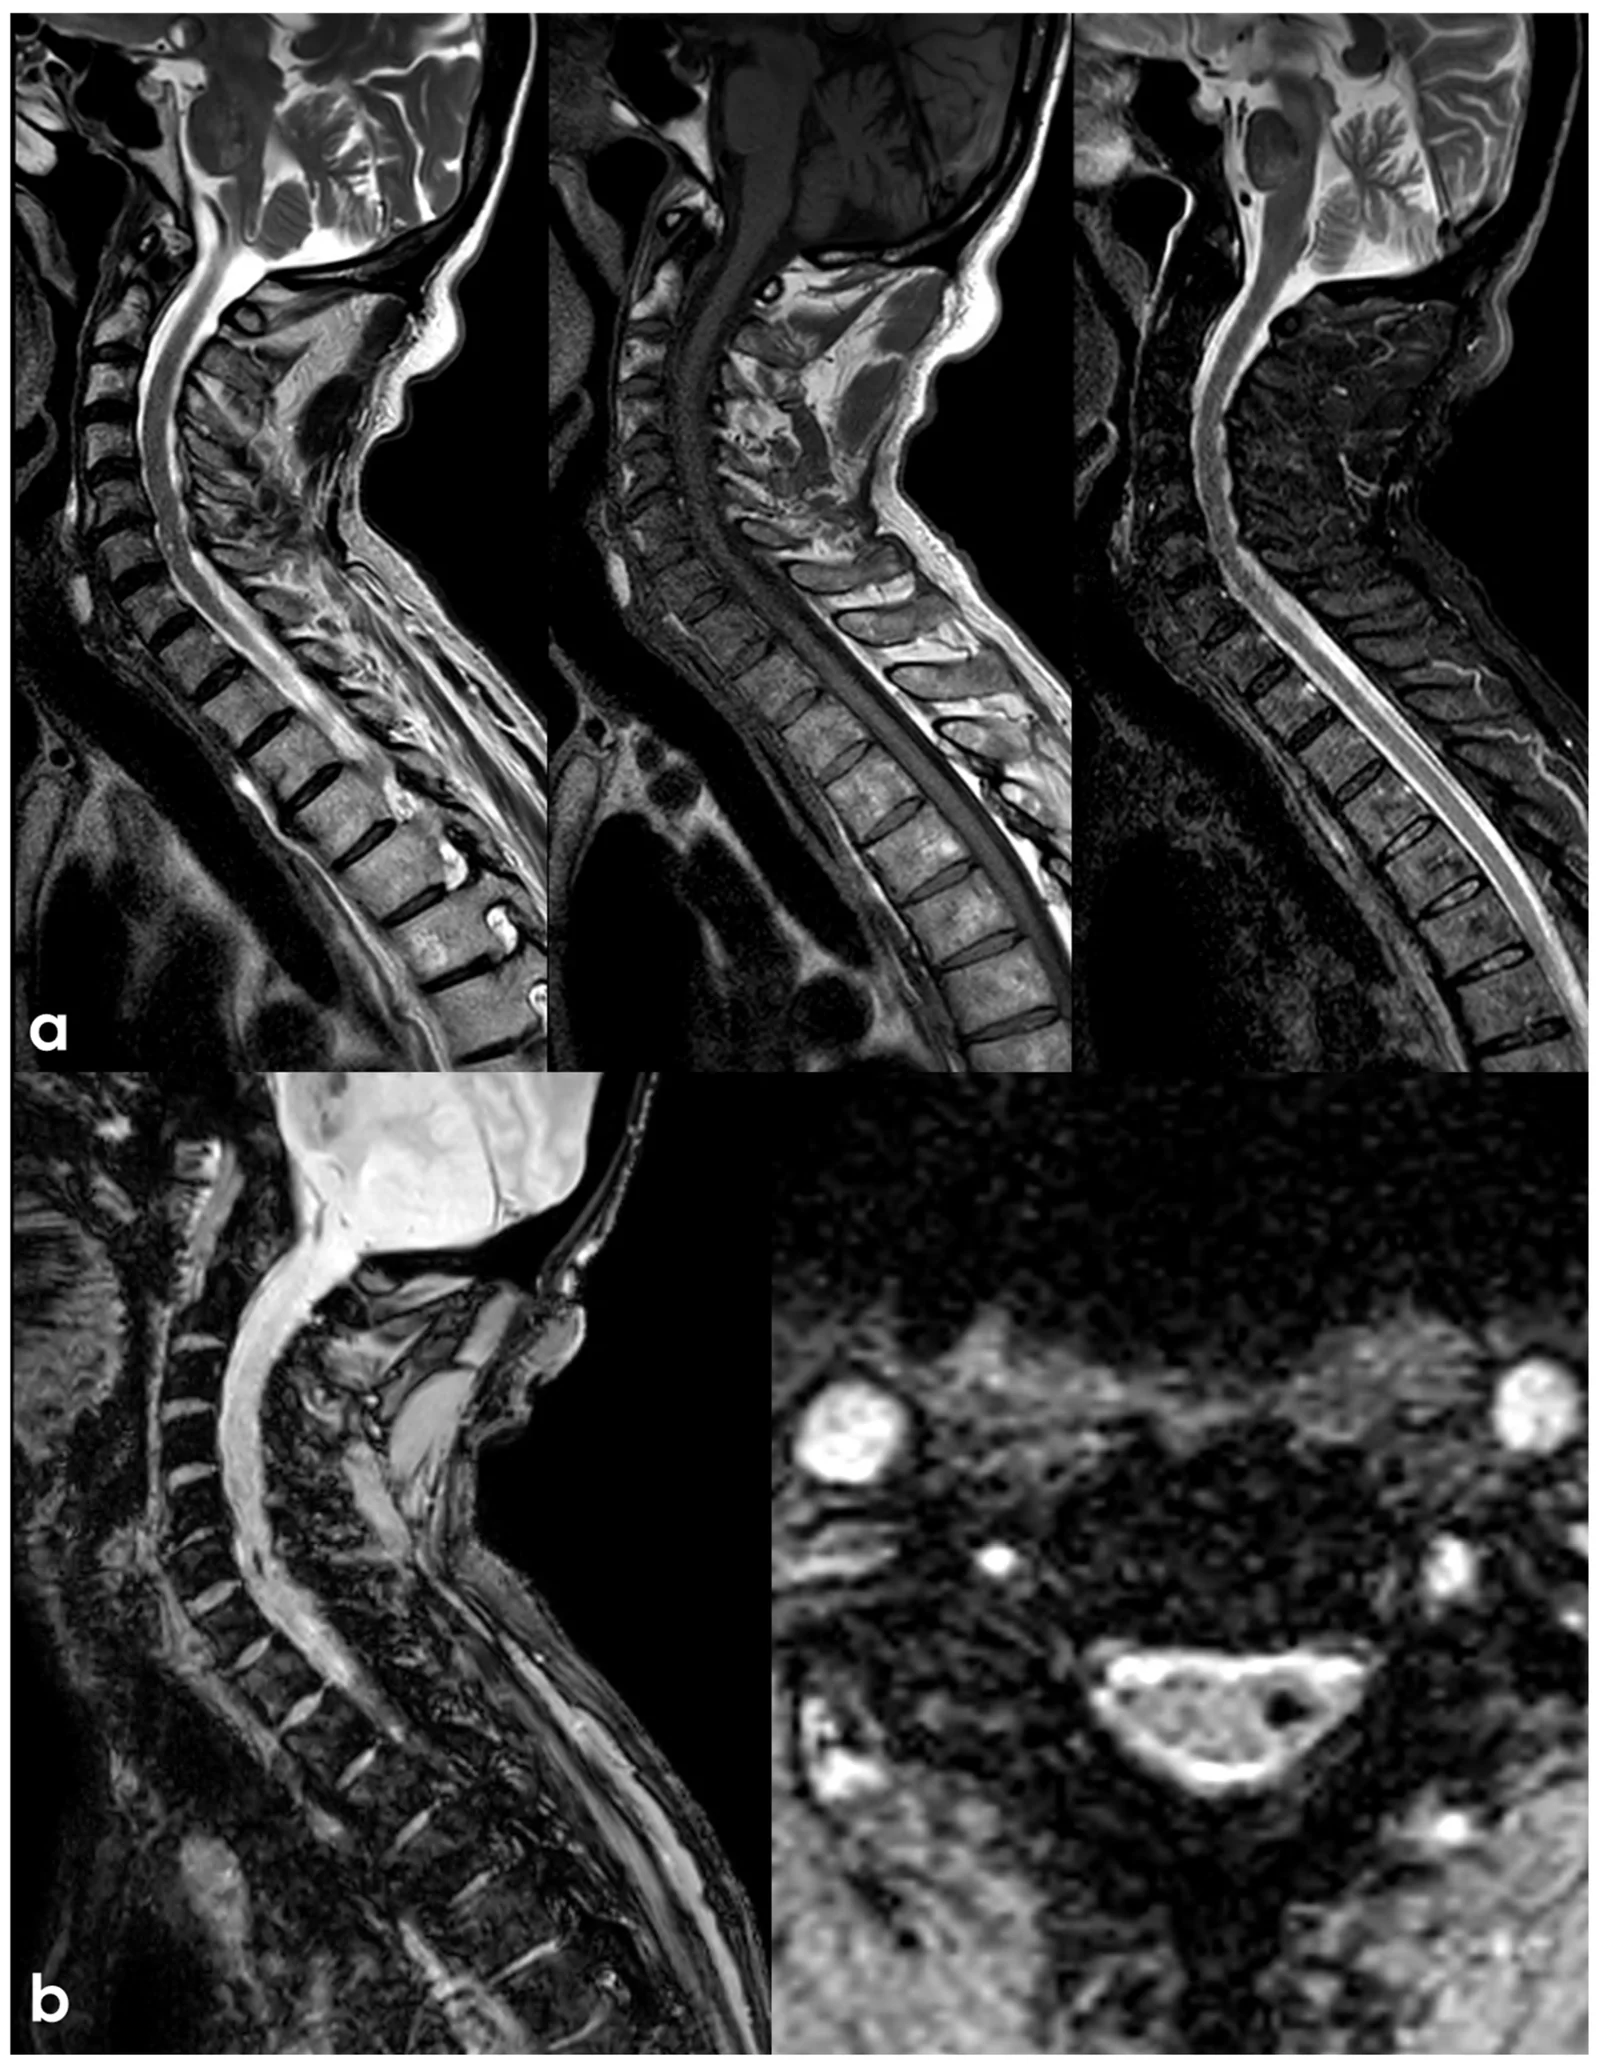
Cervical spinal MRI in the same patient of Figure 3, performed as screening for asymptomatic SCCMs. (Panel a): Sagittal T1W, T2W and STIR sequence (from (left) to (right)), showing a small rounded intramedullary lesion, with a dotted Y1W hyperintensity. (Panel b): GRE sequence in sagittal (left) and axial (right) acquisition, showing a correspondent hypointensity on the left lateral funiculus, consistent for SCCM.
